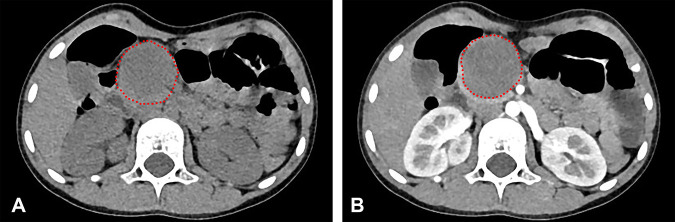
(Case 2): Non-contrast CT reveals a cystic hypodense lesion in the pancreatic head (a, red circle); on contrast-enhanced CT, it shows no enhancement with well-defined margins, and the surrounding pancreatic parenchyma exhibits a “ball-holding” sign of envelopment (b, red circle). Copyright by Chen Y, et al. [21].
